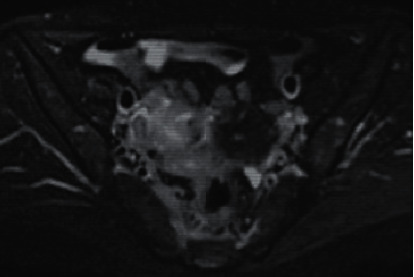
MRI image after artifact elimination based on phase correction algorithm.
